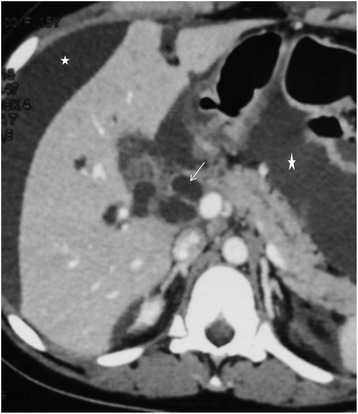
Axial computed tomography showing a dilation of the main bile duct (*arrow*) and a peritoneal fluid effusion (*asterisk*)
